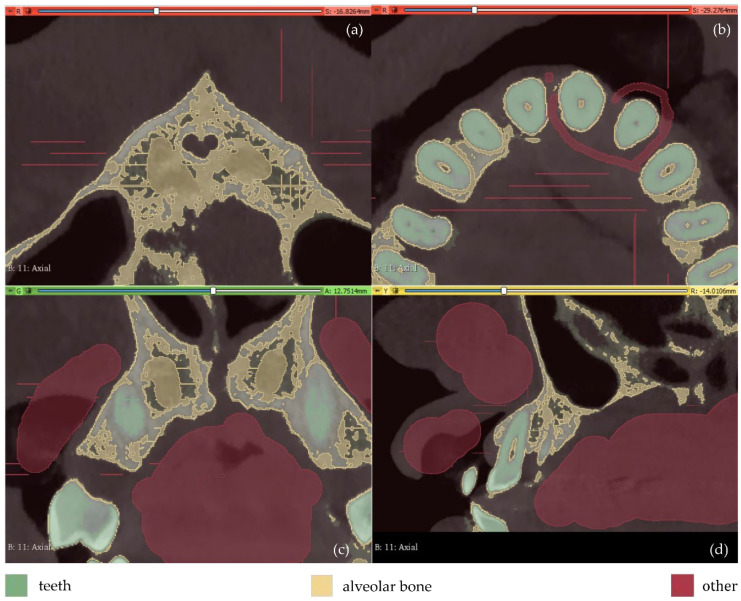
Manual definition of segments in CBCT slices (Experiment 4); (a,b): axial plane; (c): coronal plane; (d) sagittal plane.
